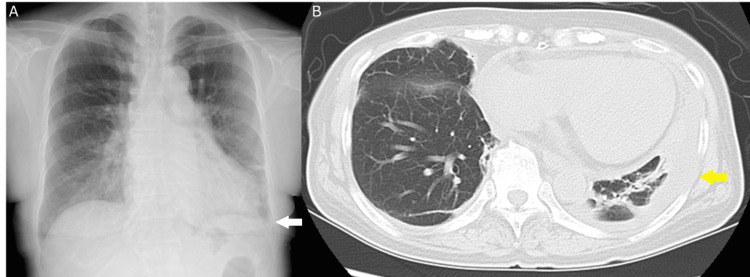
Chest X-ray and non-contrast-enhanced computed tomography (CT) of the lung (A) The chest X-ray image shows pleural effusion in the left lung. (B) The chest CT scan shows pleural effusion in the left lung.
